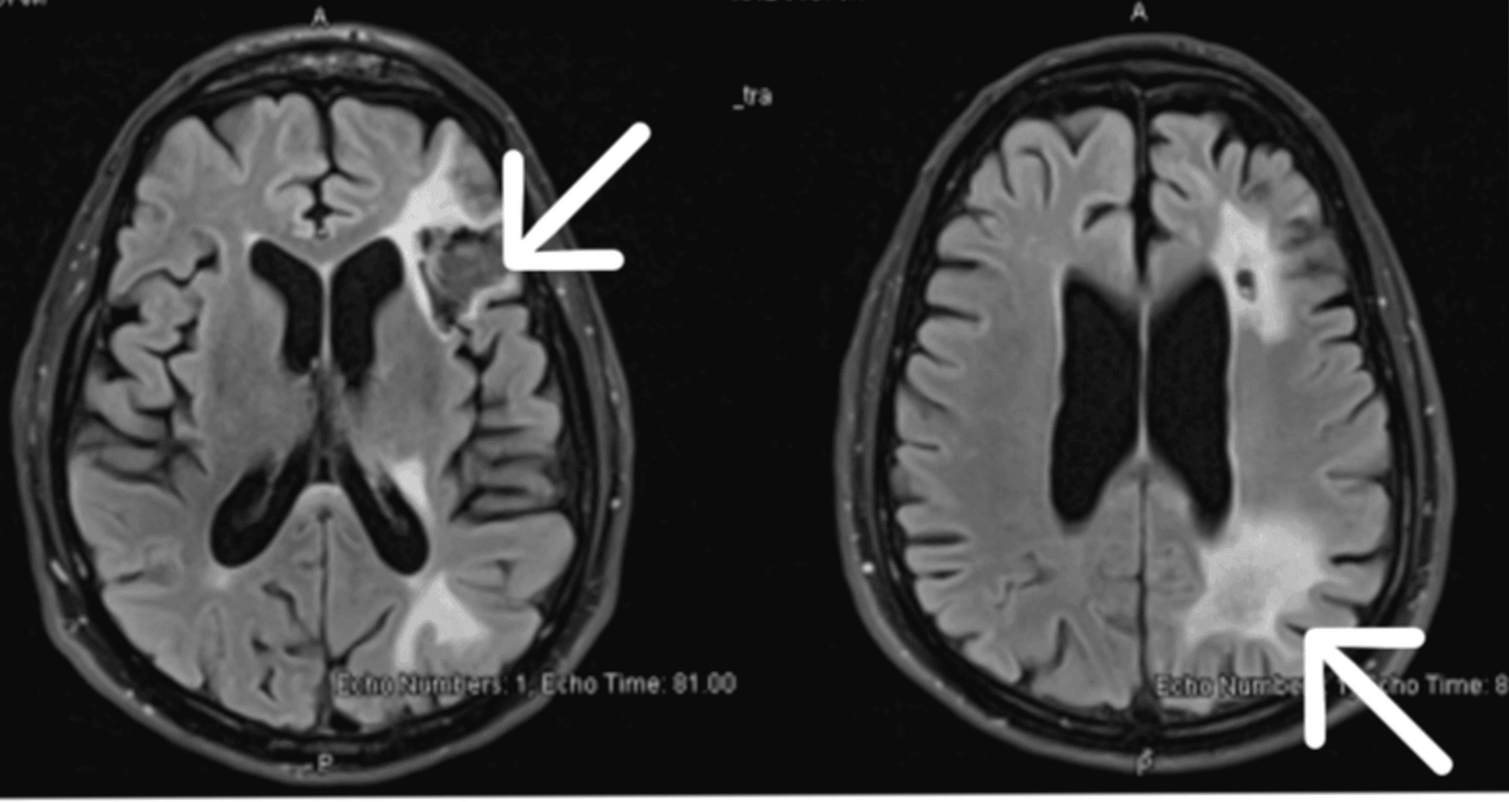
MRI brain three weeks later
